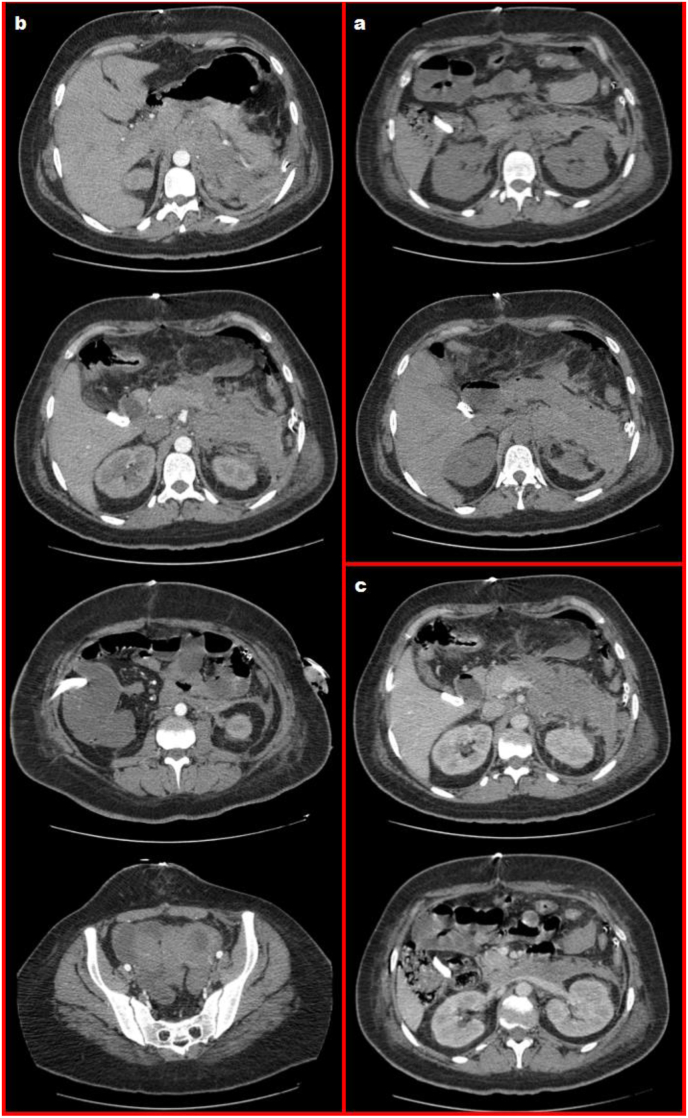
Axial C- (a), C+ arterial phase (b), and C+ venous phase (c) abdominal CT sequences showing: Spontaneously Hyper intense collection in the bolsa omental facing the pancreatic tail without contrast media extravasation in C+ sequences, Infiltration of the mesenteric fat facing the pancreatic body spontaneously hyper intense, Redon drain within the (empty) splenic lodge without contrast media extravasation in C+ sequences, Delbet drain within the hepatic hilum, Intra-peritoneal effusion of low abundance within the Morrison's pouch, the left peri-colic gutter, and in the pelvis, Poly-lobed gravid uterus.
